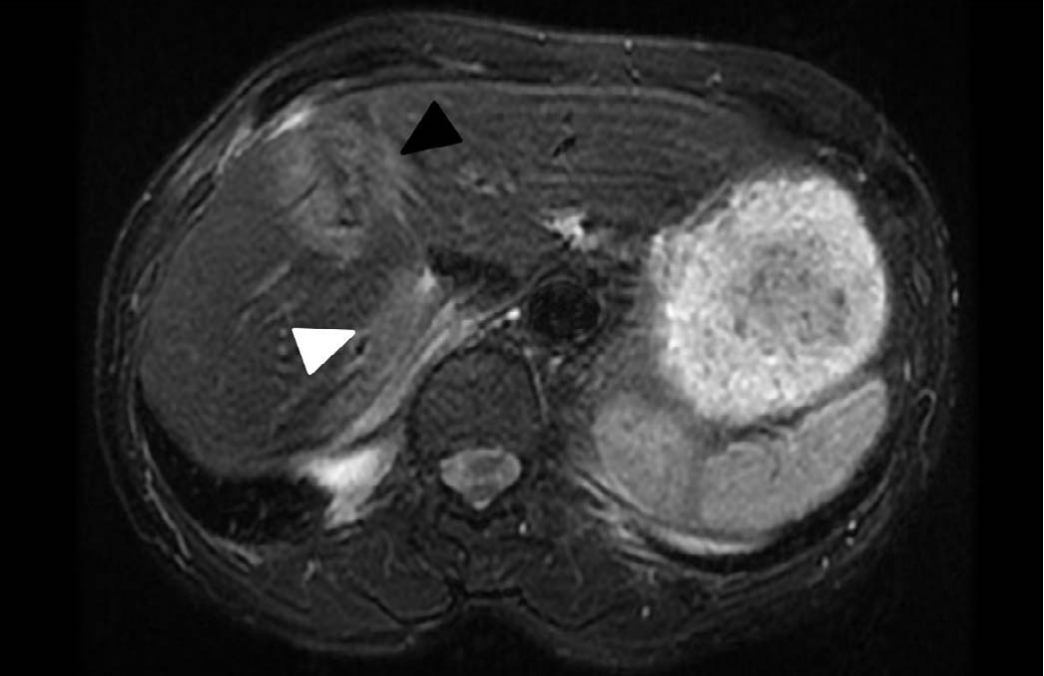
Liver magnetic resonance imaging (axial T2 FRFSE FS POST) at 1.5 years after therapy, which shows stable posttreatment changes at the original anterior tumor location (black arrow). In addition, the proton entry track of the posterior lesion is visible (white arrow). Note an area of normal-appearing liver tissue between the 2 regions with posttreatment changes.
